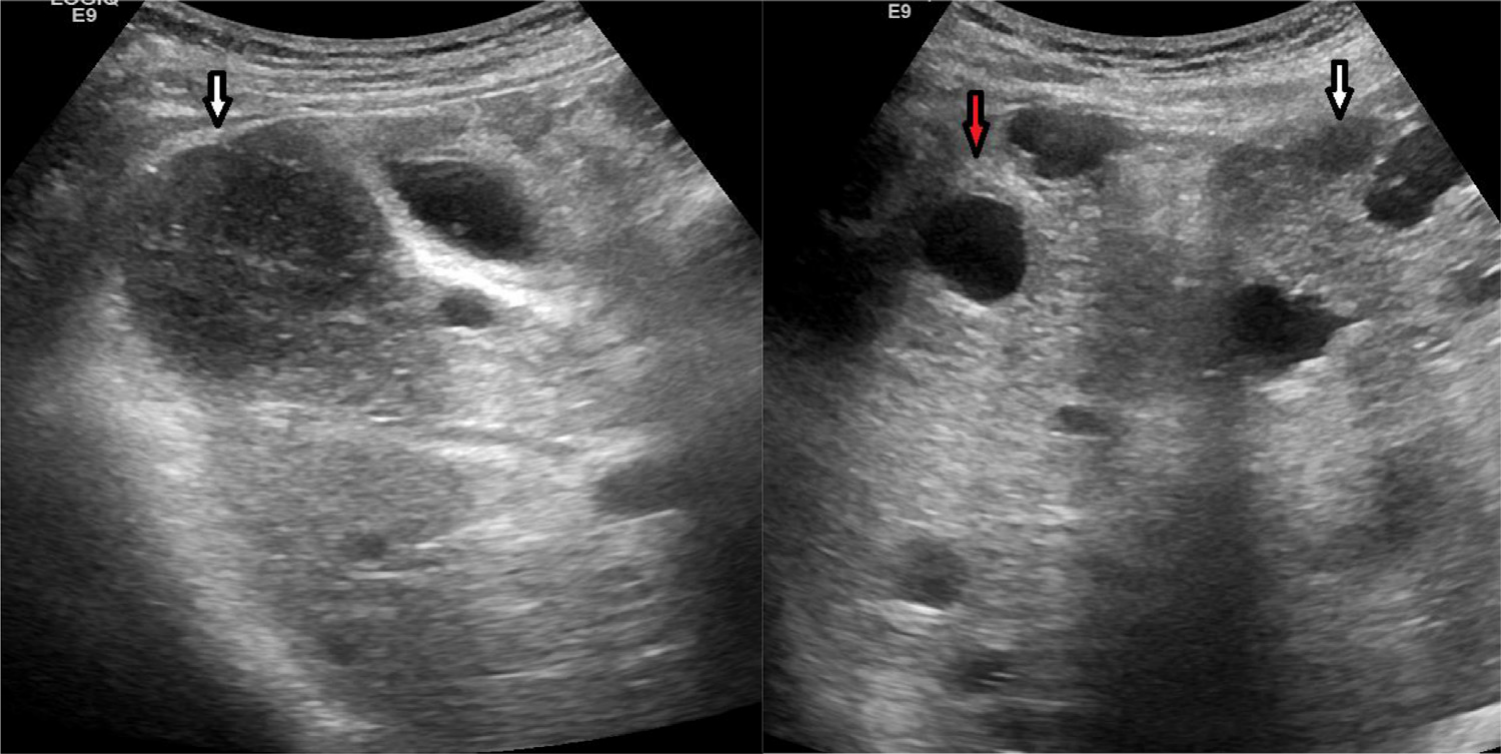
hepatic ultrasound MRI showing multiple cystic lesions (red arrow) associated to hypoechoic nodular lesions (white arrow). (Color version of figure is available online)
